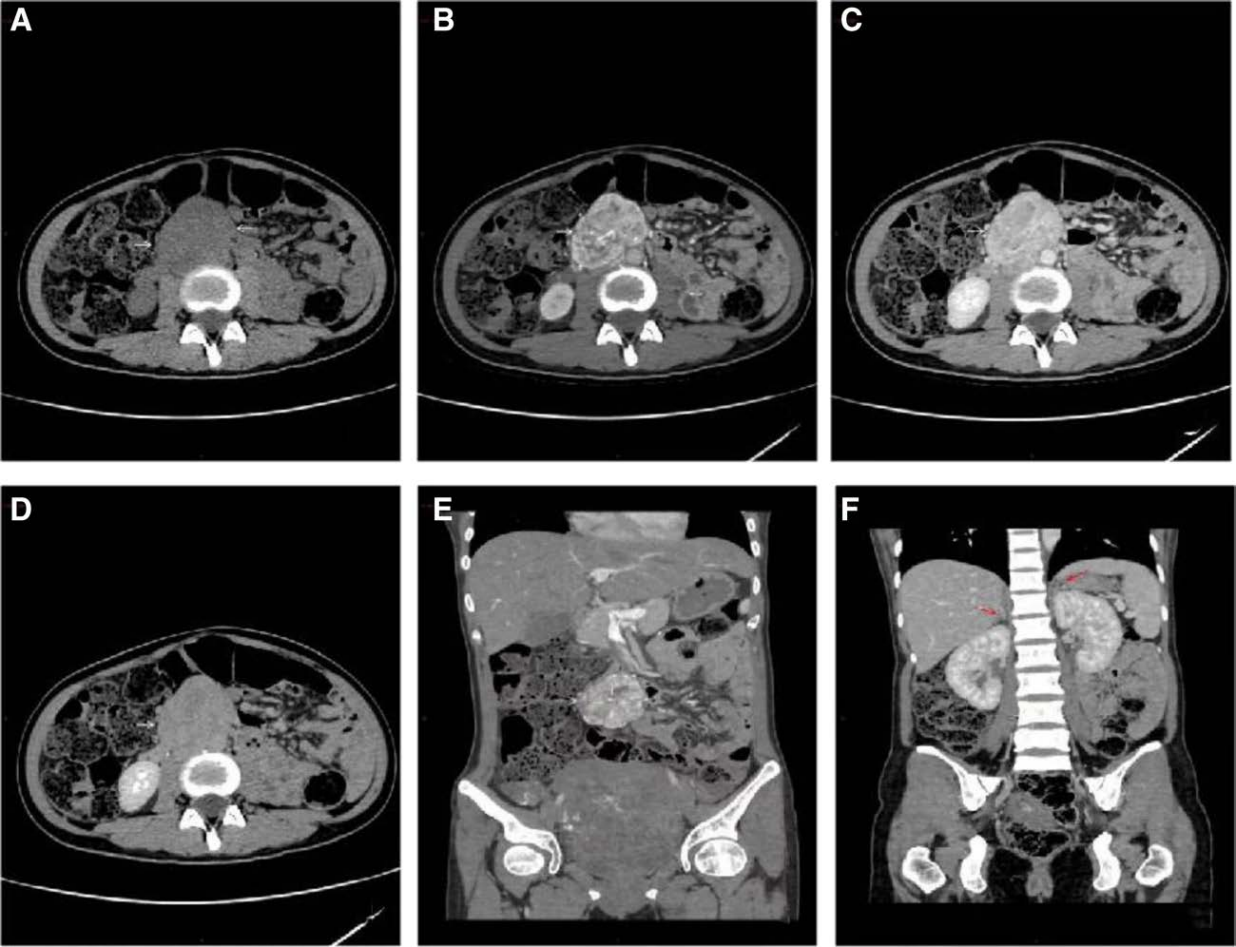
Postpartum CT scan plus enhancement scan in the entire abdomen. (A) In the axial-positioned abdominal CT image, a soft tissue density mass on the right anterior the abdominal aorta (at the very bottom of the left kidney that levels to the L4 spine) was visualized (as noted by the white arrow). The mass, measuring 6.0 cm × 4.5 cm × 6.6 cm, had a clear boundary that gradually turned to a shallow lobe. The CT value was 35HU. (B, C, D, E) In the enhanced three-phase and axial-positioned abdominal CT scan images, and the enhanced portal venous phase and coronal-positioned images, enhancement scan results at the abdominal aorta indicated that irregular changes were markedly observed in the mass, which enhanced significantly in arterial phase but reduced in portal venous phase and equilibrium phase. The CT value was 135HU, 92HU and 60HU in respective. Inside the abdomen seen a weakly enhanced small patches (as noted by the white arrow). (F) In the coronal-positioned enhanced CT scan image of portal venous phase, the shapes and density of the junction line between the 2 glands and their middle and lateral limbs showed no notable abnormality (as noted by the red arrow). CT: computed tomography.
